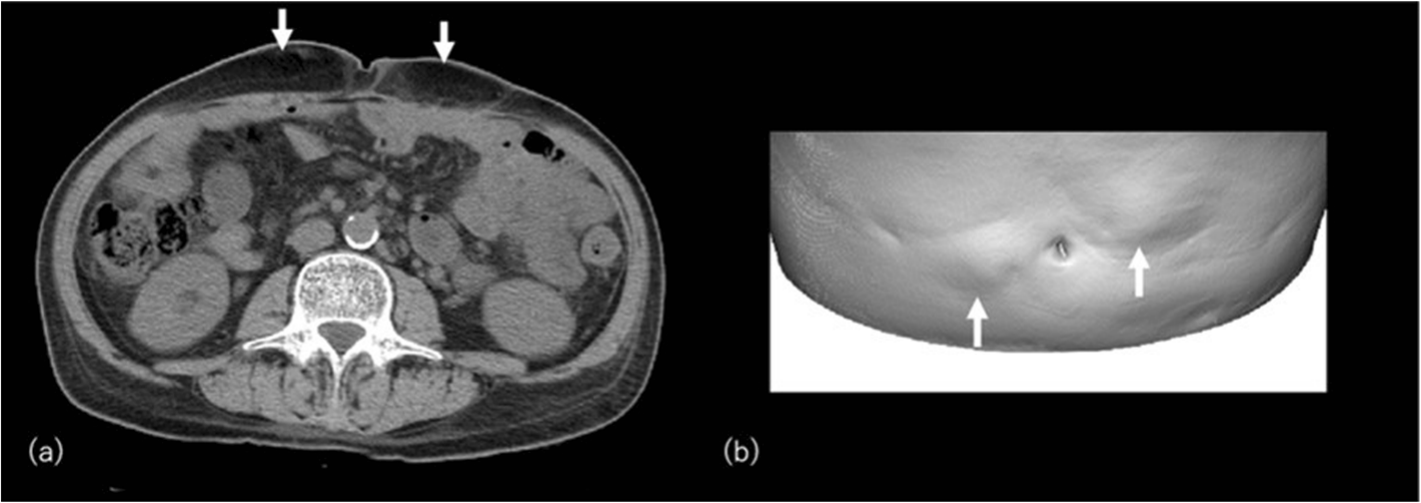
A 66-year-old man with type I diabetes and insulin injection lipohypertrophy. a CT image at the level of belly button shows bilateral localized subcutaneous fat deposition on the anterior abdominal wall (arrows). b Volume-rendered three-dimensional reformation image show bilateral bulging of abdominal wall reflecting the insulin lipohypertrophy
